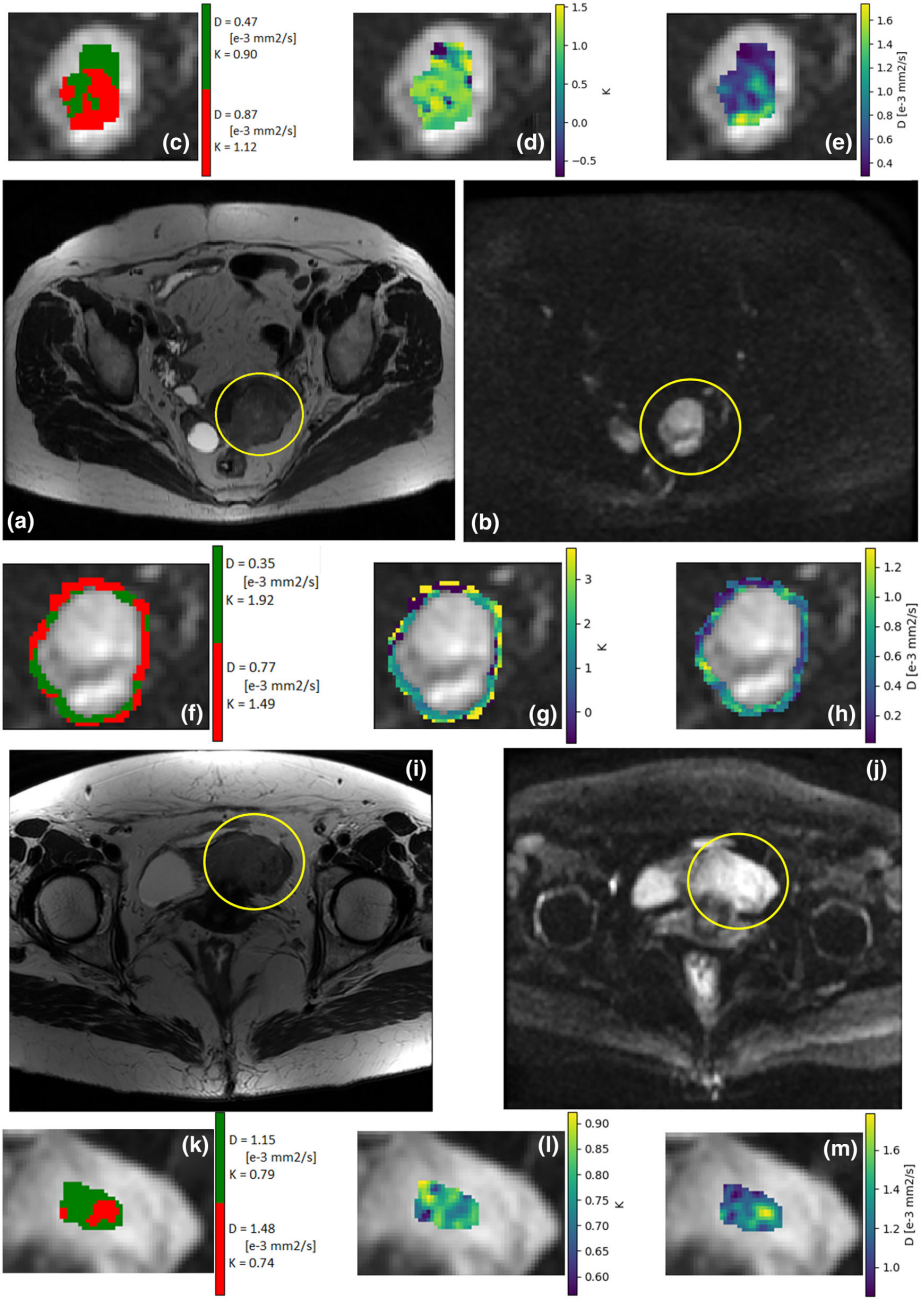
(a) 73‐year‐old patient with grade 1 (G1) endometrioid adenocarcinoma. a) the T_2_ image shows a slightly higher signal intensity in cancer. (b) a diffusion‐weighted image, DWI (b value = 500 $s/mm^{2}$); (c) DWI shows the clusters’ labels on the tumoral ROI. (d) the parametric map of the diffusion coefficient D shows a low value of D within the tumor. (e) parametric map of the kurtosis coefficient K shows a high value of K in the T ROI; (f) clusters’ labels on the peritumoral ROI; (g) D map on the PT ROI; (h) K map on the PT ROI; (i) T_2_ image of a 65‐year‐old healthy subject; (j) DWI (*b*‐value = 500 $s/mm^{2}$); (k) clusters’ labels on the healthy ROI; (l) D map on the H ROI; (m) K map on the H ROI. ROI, region of interest
